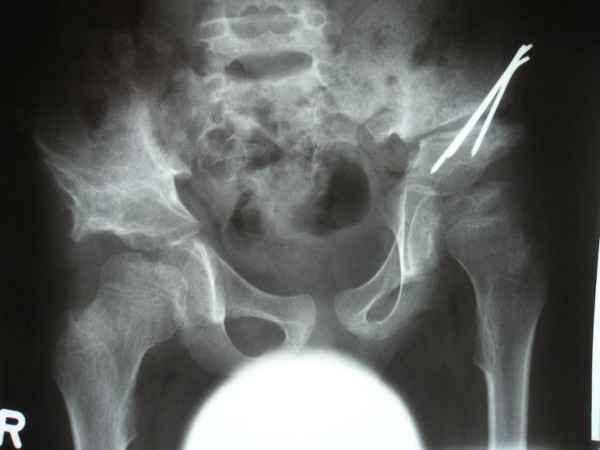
Anteroposterior pelvis radiograph showed irregular ossification of the epiphyses. Defective ossification of the femoral heads (significant flattening of the capital femoral epiphyses associated with widened femoral necks, dysplastic acetabulae and coxa valga. At this age (six years) a varus derotation osteotomy and a Salter osteotomy was performed.
